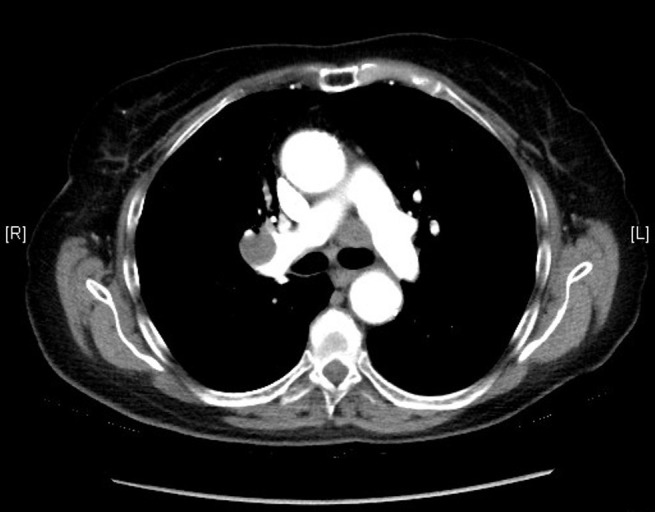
CT scan of the chest from 2015 showing right hilar adenopathy
